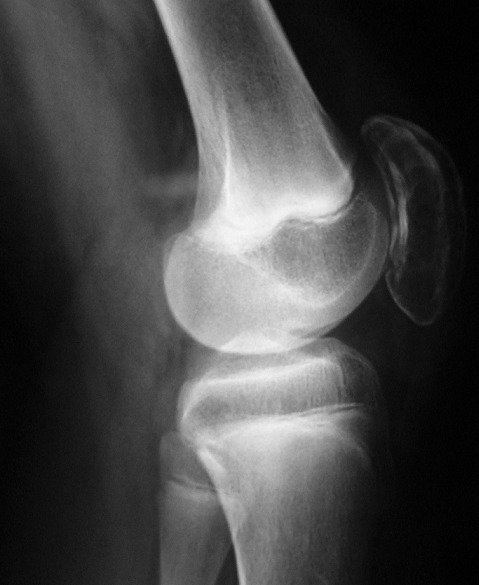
Radiography of the right knee showing an osteolytic lesion involving the hole patella
